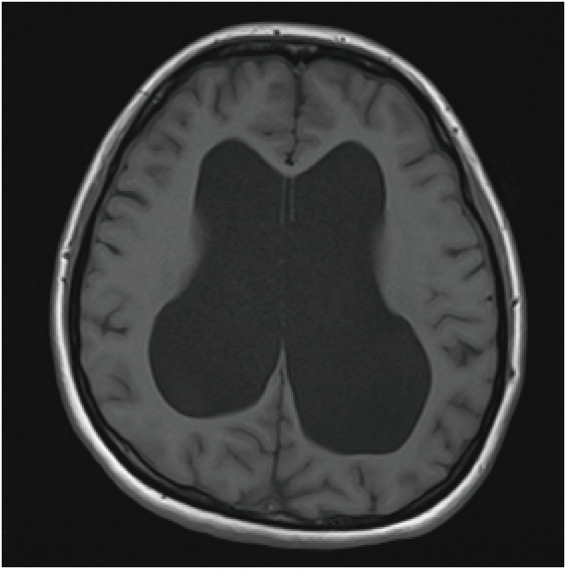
Representative axial brain MRI demonstrating hydrocephalus. Axial T1-weighted MRI image shows marked, symmetric dilatation of the bilateral lateral ventricles with thinning of the surrounding cerebral mantle, consistent with severe ventriculomegaly/hydrocephalus.
